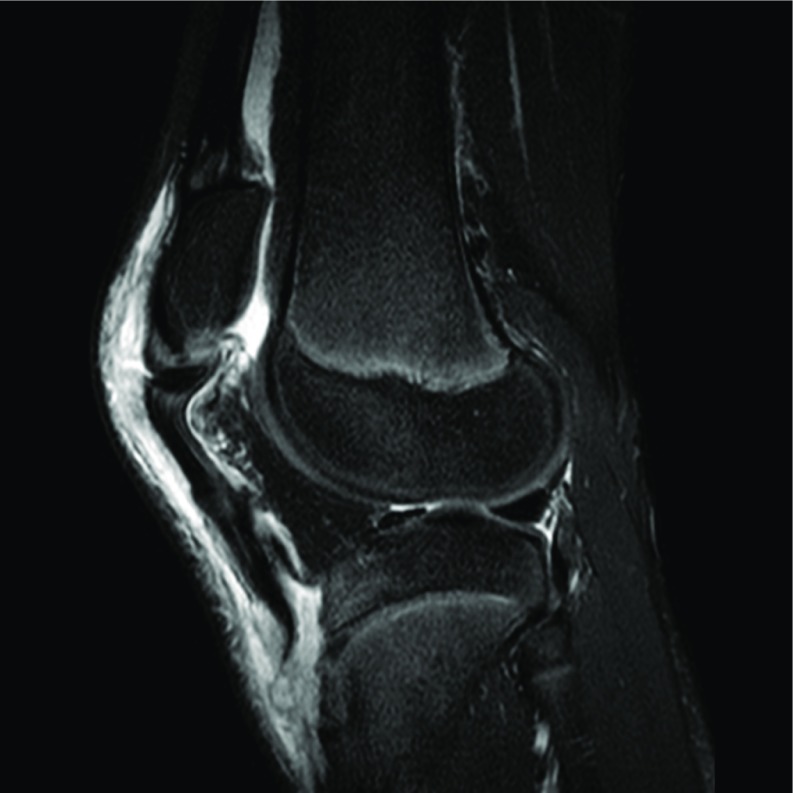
Preoperative sagittal MRI demonstrating bifocal avulsion sleeve fracture from both distal patella and tibial tubercle as described in Case 2.
